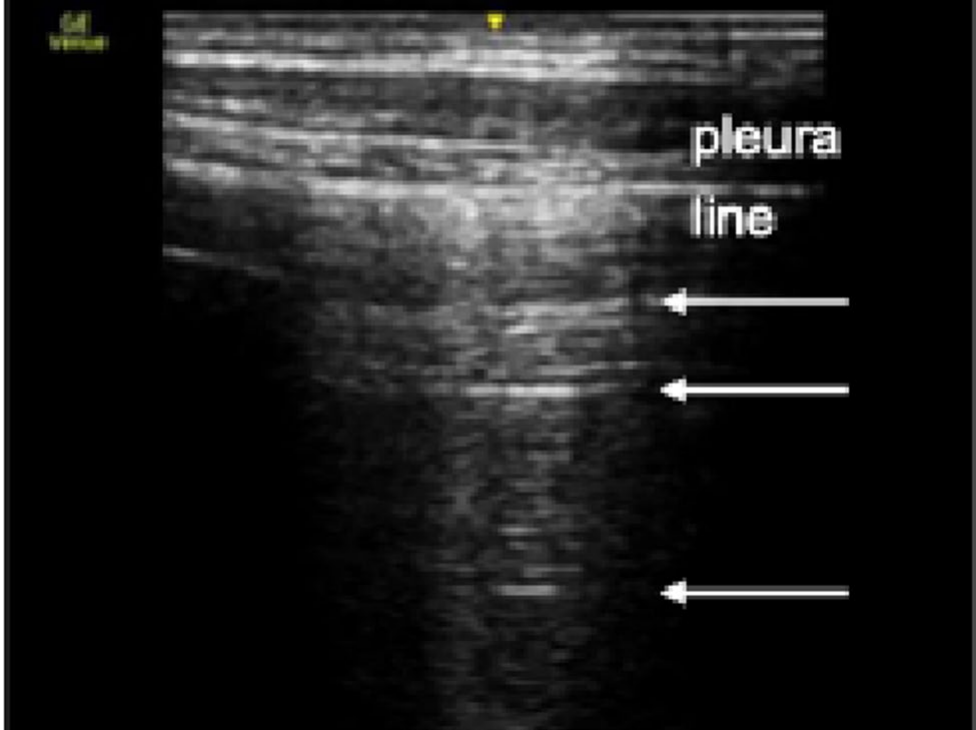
Ultrasound image of A-lines. Arrows indicate A-lines
